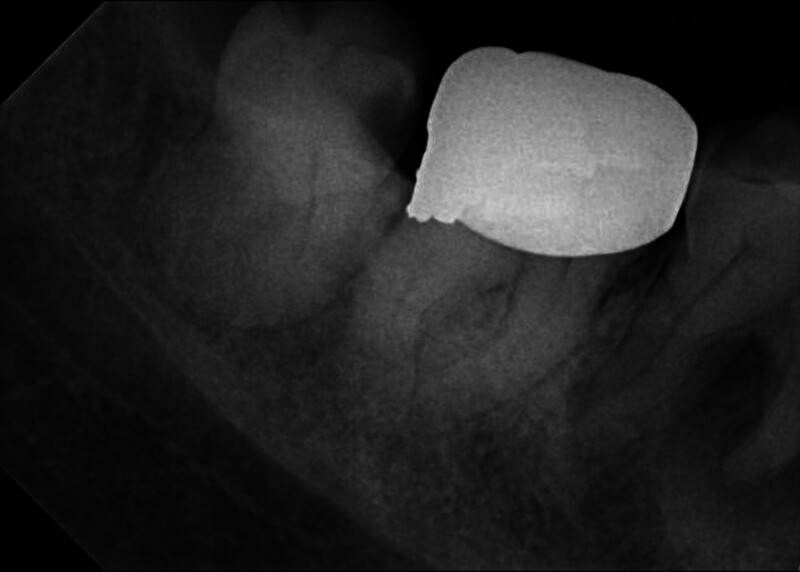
Digital periapical radiograph: the mesial part of the crown of the right mandibular third molar is defected and close to the pulp cavity, the crown of the second molar shows the appearance of a metal crown, and the periapical area of the first molar shows a patchy low-density shadow with an unclear boundary (the low-density shadow is larger compared with the oral panoramic radiograph in December 2014).
